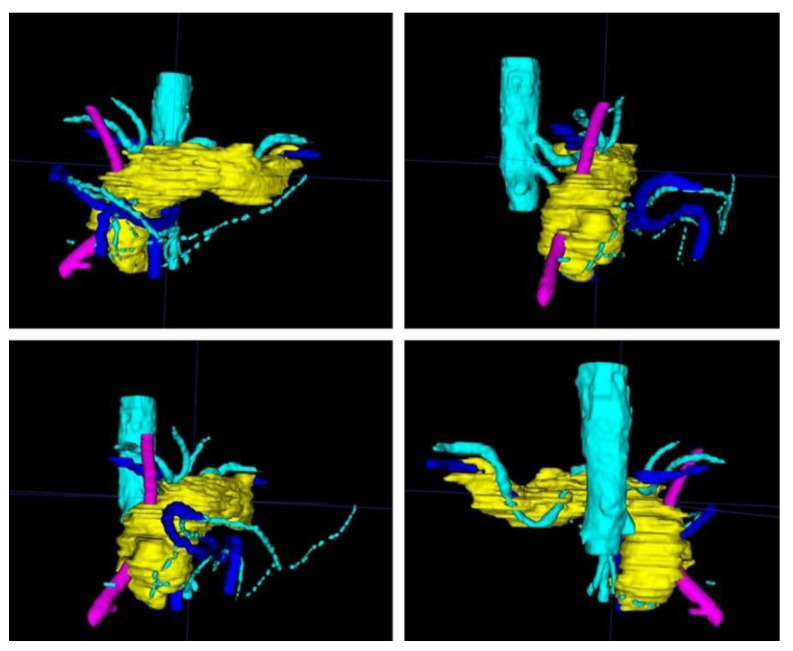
After completion of the final mesh, the pancreas and its surroundings can be viewed from different angles. Note the rather rough surface as the CT scans had a slice thickness of 1 mm. (The pancreas in yellow, the arteries in light blue, the veins in dark blue, and the splint in pink.)
